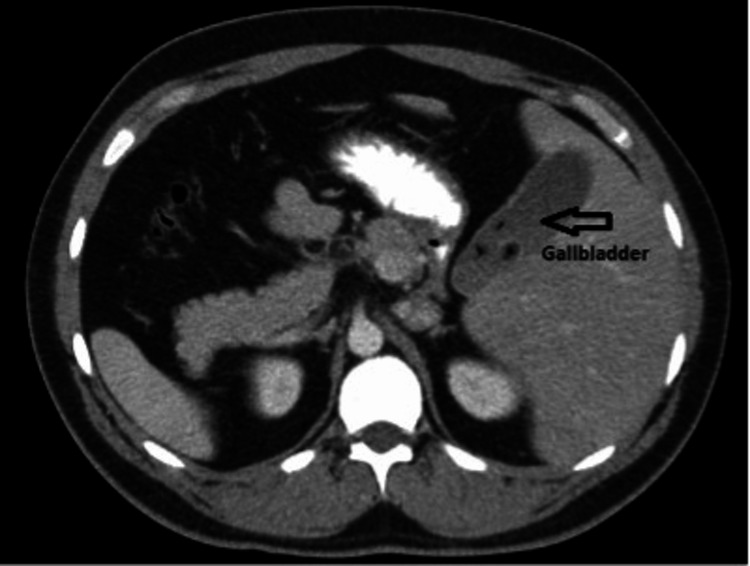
CT scan of the patient with SI. Stones in the gallbladder can be seen The surgeon was on the patient's right side and the assistant on the patient's left side
